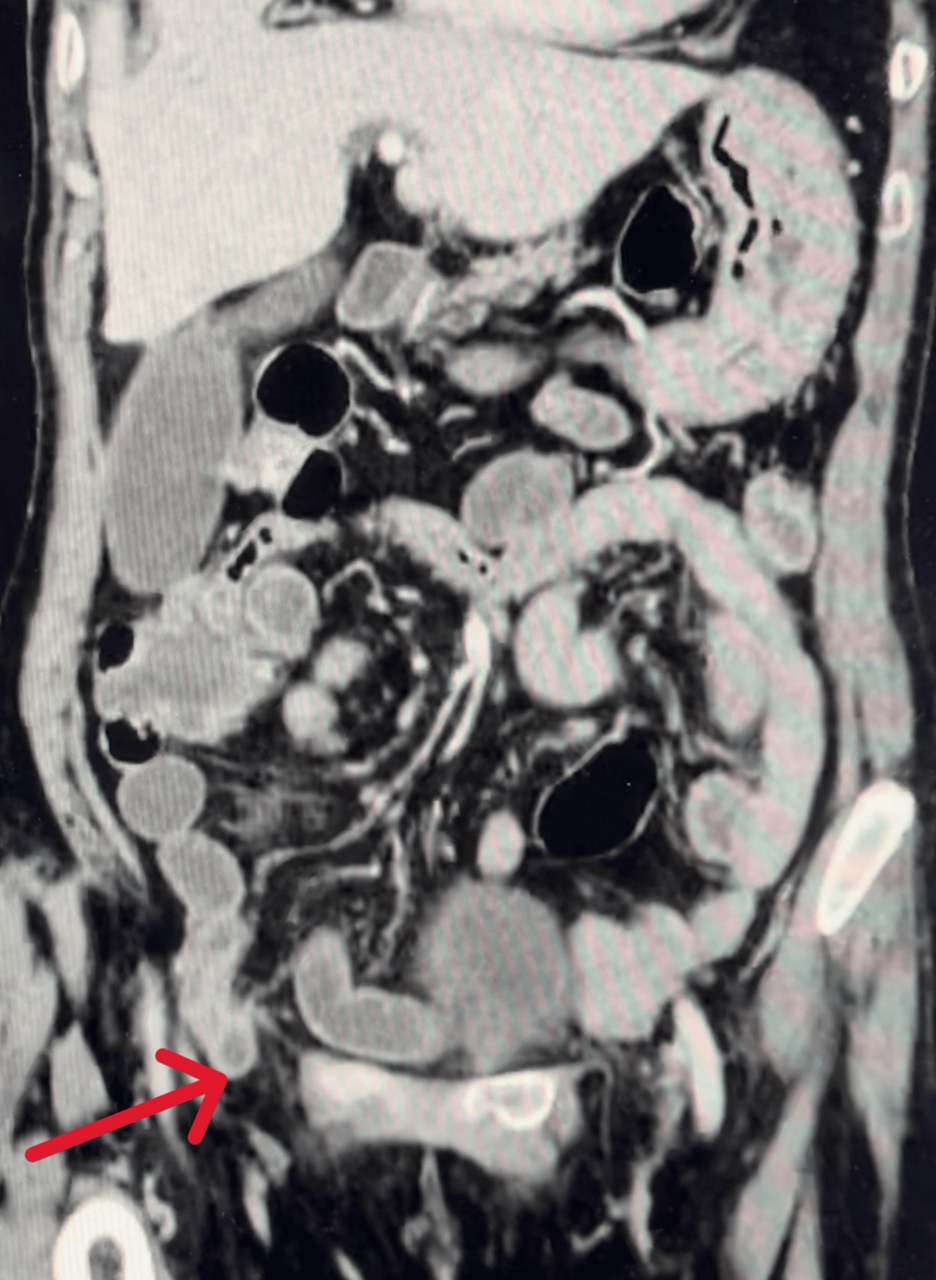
Abdominal CT scan showing a right obturator hernia, with the small intestine component highlighted by the red arrow (frontal plane)
